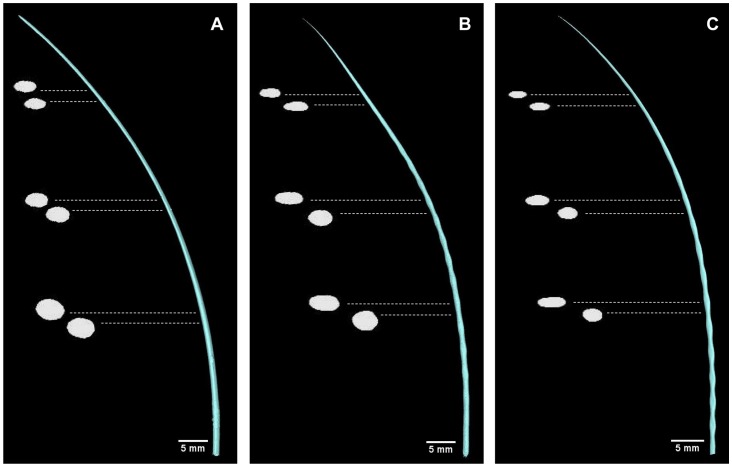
Comparative digital cross-sections from CT data. Reconstructions of vibrissae from CT scan data. (A) California sea lion; (B) elephant seal; (C) harbor seal. Enlarged digital cross-sections are shown at six points along the whisker length. Scale bar represents scaling for whole whisker image. Cross-sections are approximately 4–5x enlarged. In smooth vibrissae, the cross-sectional shape is consistent between neighboring points along the shaft, while undulated vibrissae vary in cross-sectional shape between troughs to crests. The cross-sections of all vibrissae show increased flattening toward the tip.
